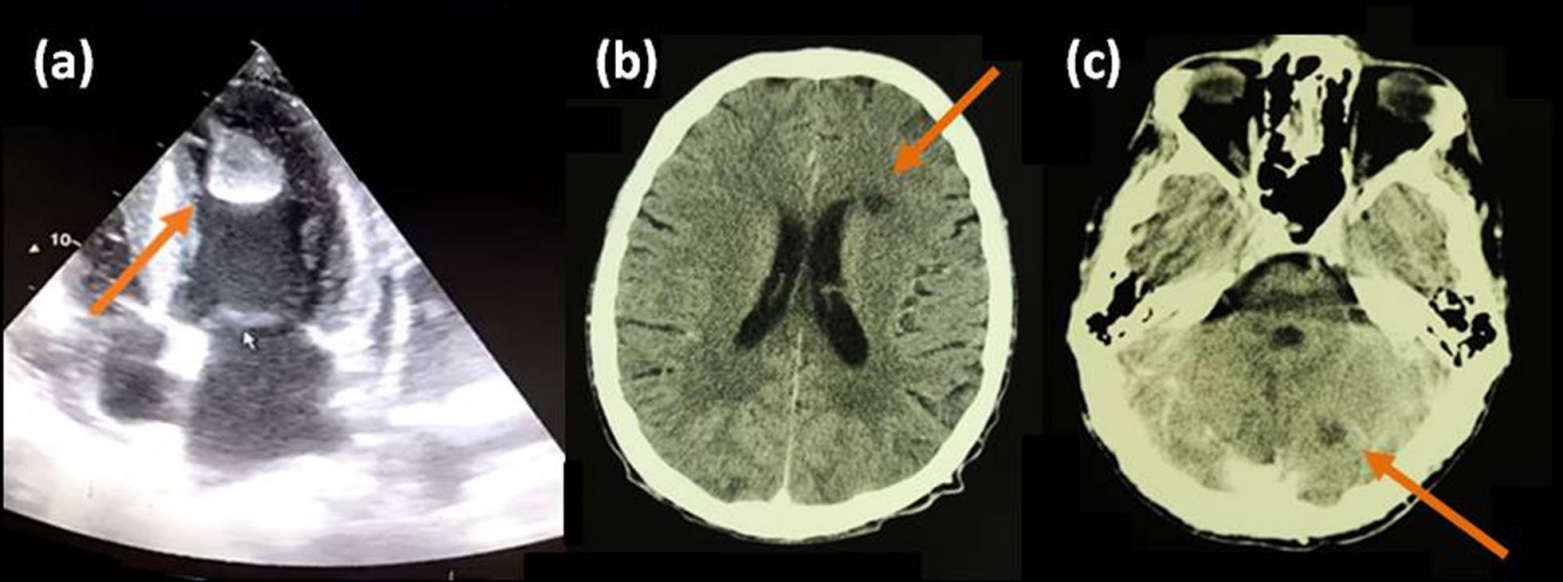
Pre-IVT TTE exhibiting LV thrombus (3.5 × 2.5 cm) with a narrow stalk (a) and CT scan demonstrating an ovoid hypodense lesion located in the vicinity of the left frontal horn (paraventricular white matter) (b) and a second similar hypodense lesion located in the left cerebellar hemisphere (c). Both CT lesions are imaging-wise compatible with lesions of a late subacute AIS-phase
